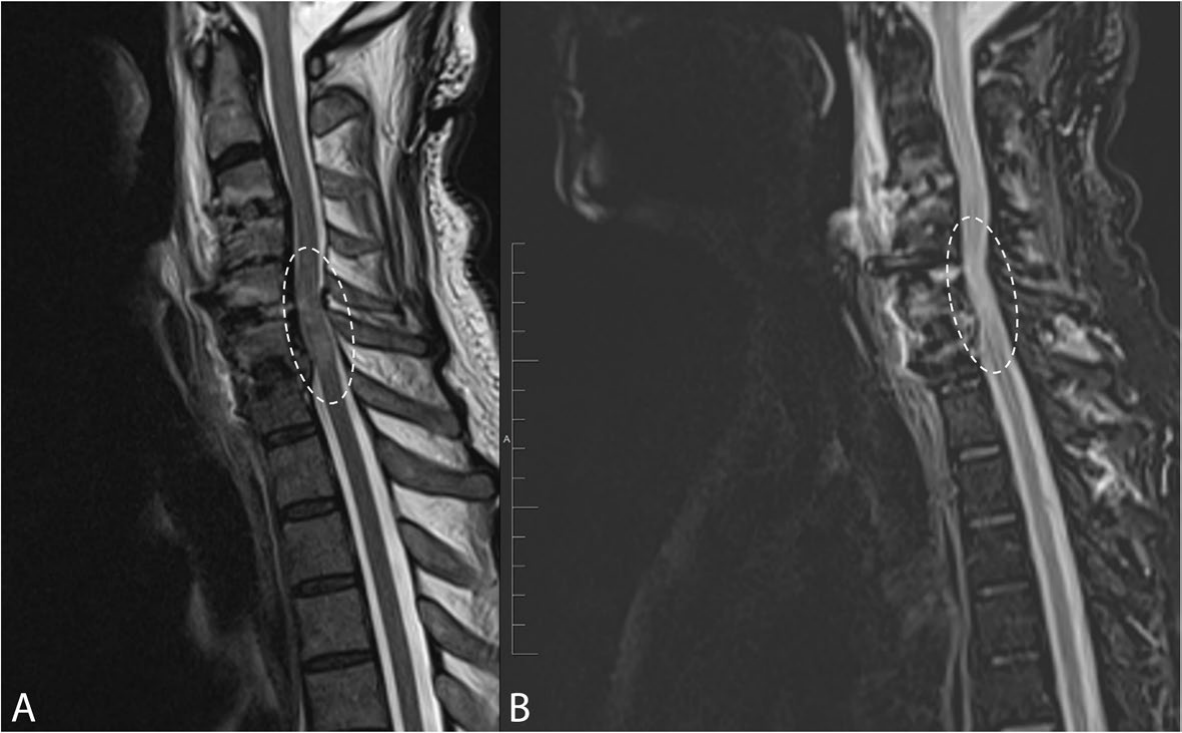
Post-operative sagittal T2 (A) and STIR (B) MRI images one month later showing regression of the previously seen high T2 signal intensity (encircled)
